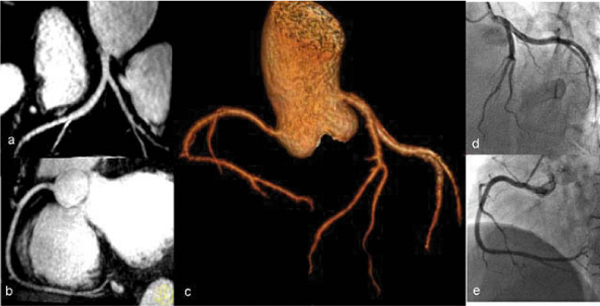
3 T Coronary MR image of a 50-year-old patient. Reformatted images (a, b) and volume rendering image demonstrates normal RCA, LM, LAD. Better visualization of the entire coronary artery tree after removing the background of myocardium, long segments of all major coronary arteries are well depicted and correlate well with X-ray angiography (d, e).
